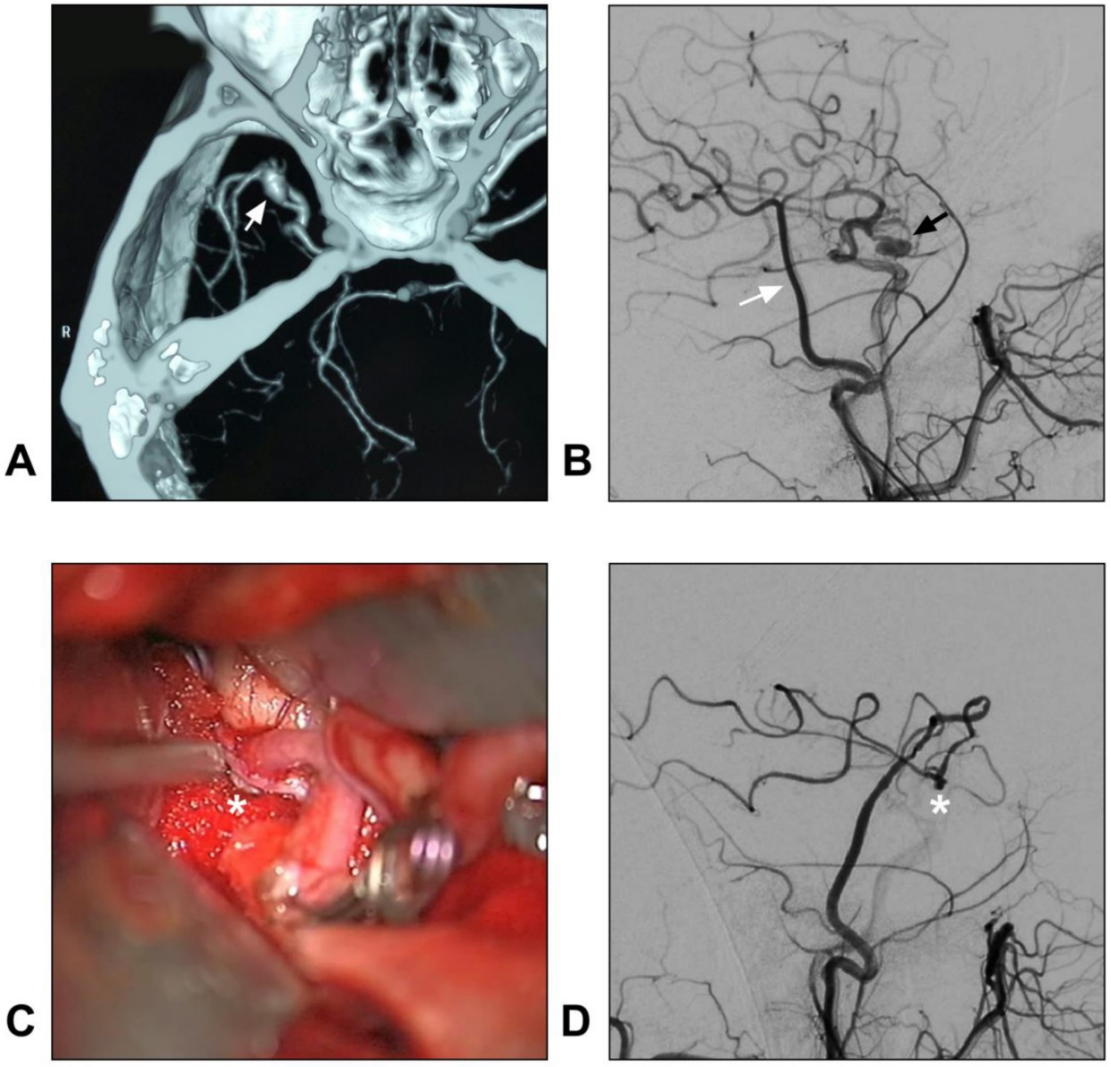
STA-MCA bypass for intracranial aneurysm. A: CTA reveals a dissecting aneurysm on the trunk of the MCA (arrow); B: DSA of the CCA shows the STA (white arrow) and aneurysm (black arrow); C: An end-to-side anastomosis between the STA and MCA trunk (asterisk) is performed; D: Postoperative angiogram of the ECA shows the STA-MCA bypass (asterisk) is patent. Abbreviations: CCA: common carotid artery; CTA: computed tomography angiography; DSA: digital subtraction angiography; ECA: external carotid artery; MCA: middle cerebral artery; STA: superficial temporal artery.
